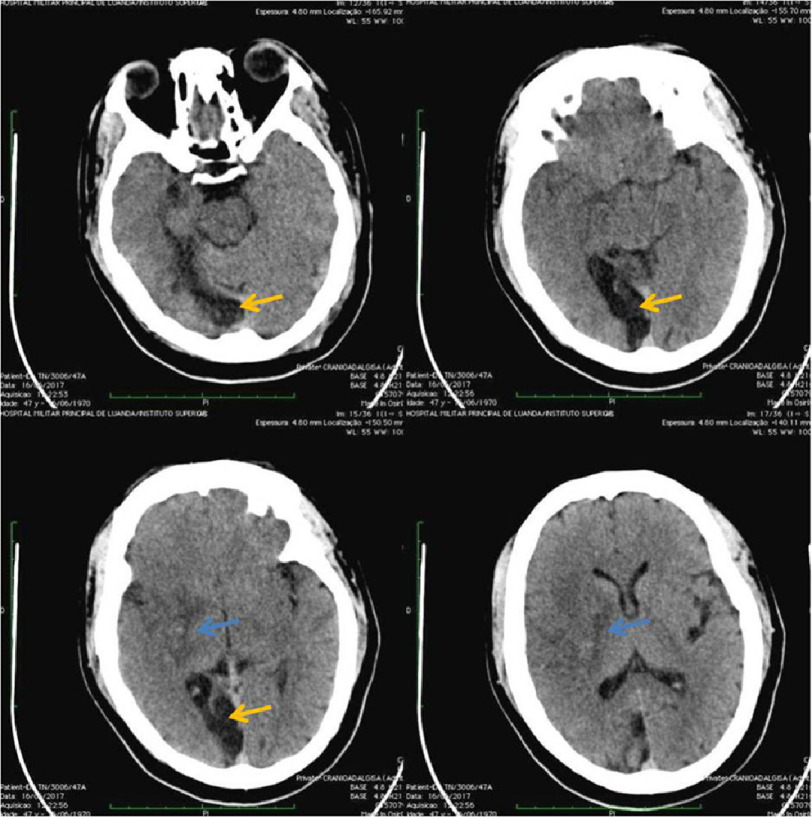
Cranioencephalic computed tomography reveals intraparenchymal hematoma undergoing resorption in the right nucleus capsular topography with surrounding edema (blue arrow); two encephalomalacia foci in the right cerebral hemisphere (yellow arrow); the findings suggest subacute stroke, coexisting foci of chronic stroke.
